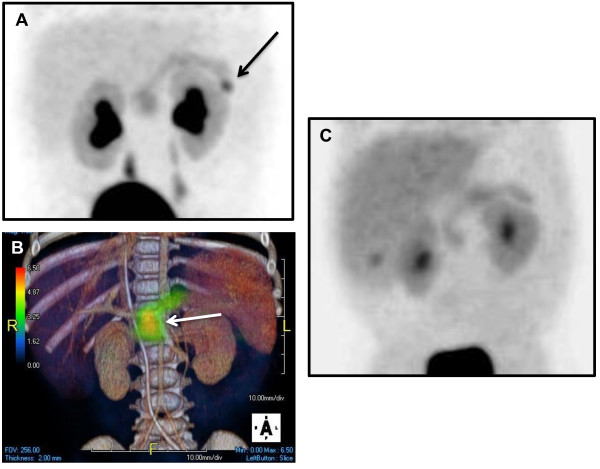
A. Frontal view of a 3D maximum intensity projection (MIP) 18-fluoro L-3,4-dihydroxyphenylalanine positron emission tomography (^18 ^F-DOPA PET) image demonstrating a focal lesion in the tail of the pancreas (arrow). B. A frontal view 3D MIP ^18^ F-DOPA PET image fused with a contrast-enhanced CT shows a focal lesion in the pancreatic head (white arrow). C. Frontal view of a 3D MIP showing non-uniform pattern of uptake with increased activity throughout the pancreas consistent with diffuse disease. Note the normal liver, kidney and bladder uptake.
